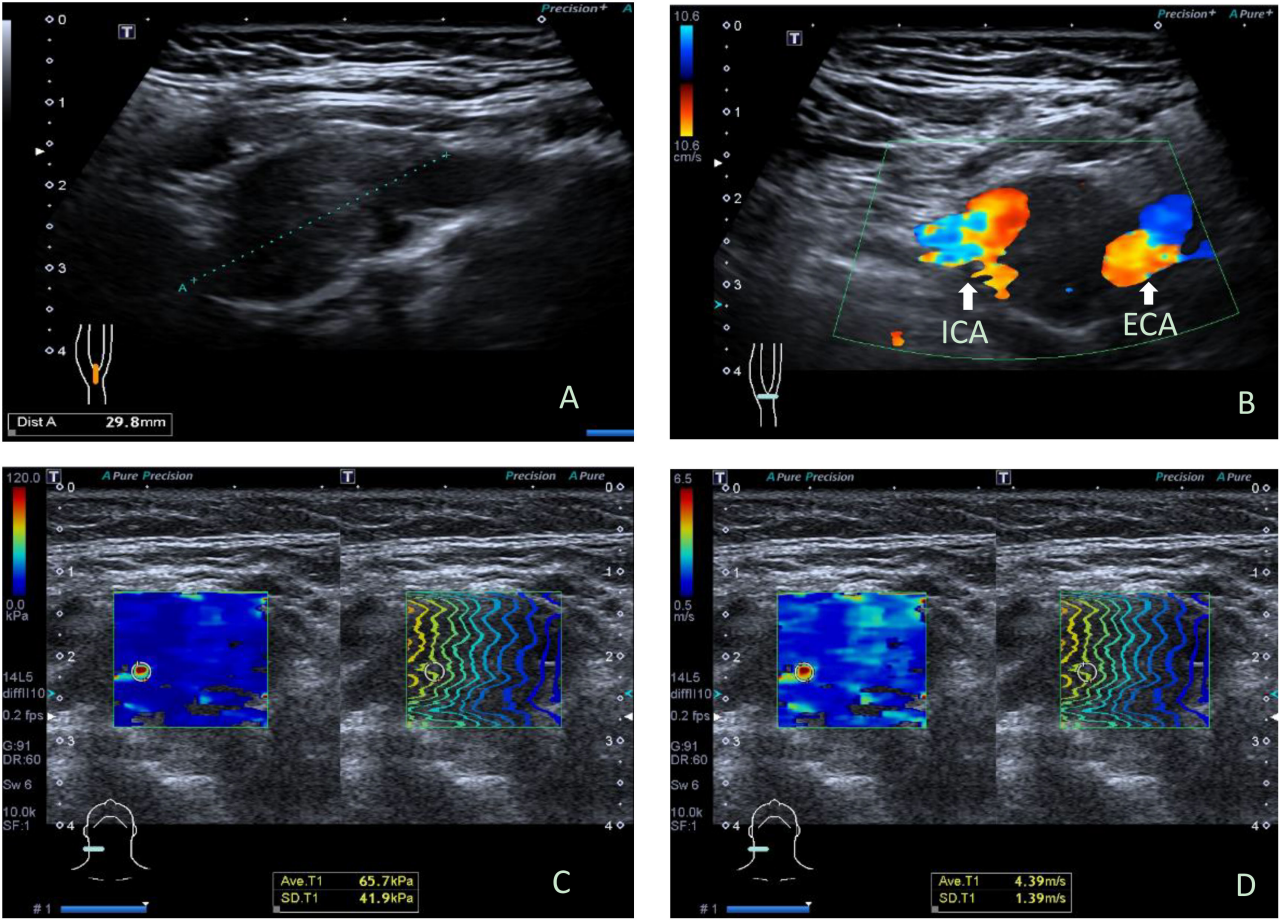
Ultrasonographic images in a 34y female with benign carotid body tumor. (A) Conventional longitudinal B-mode sonogram showed an oval hypoechoic mass with clear boundary located at the right carotid bifurcation, (B) Color Doppler flow imaging on transverse image showed the carotid body tumor partially encloses internal carotid artery and external carotid artery, splaying the carotid bifurcation. (C) The parameters of SWE on elasticity mode were as follows: Emax: 65.7kPa, SDmax: 41.9 kPa; (D) The parameters of SWE on speed mode were as follows: Emax: 4.39m/s, SDmax: 1.39cm/s. ICA, internal carotid artery; ECA, external carotid artery; SWE, shear wave elastography.
